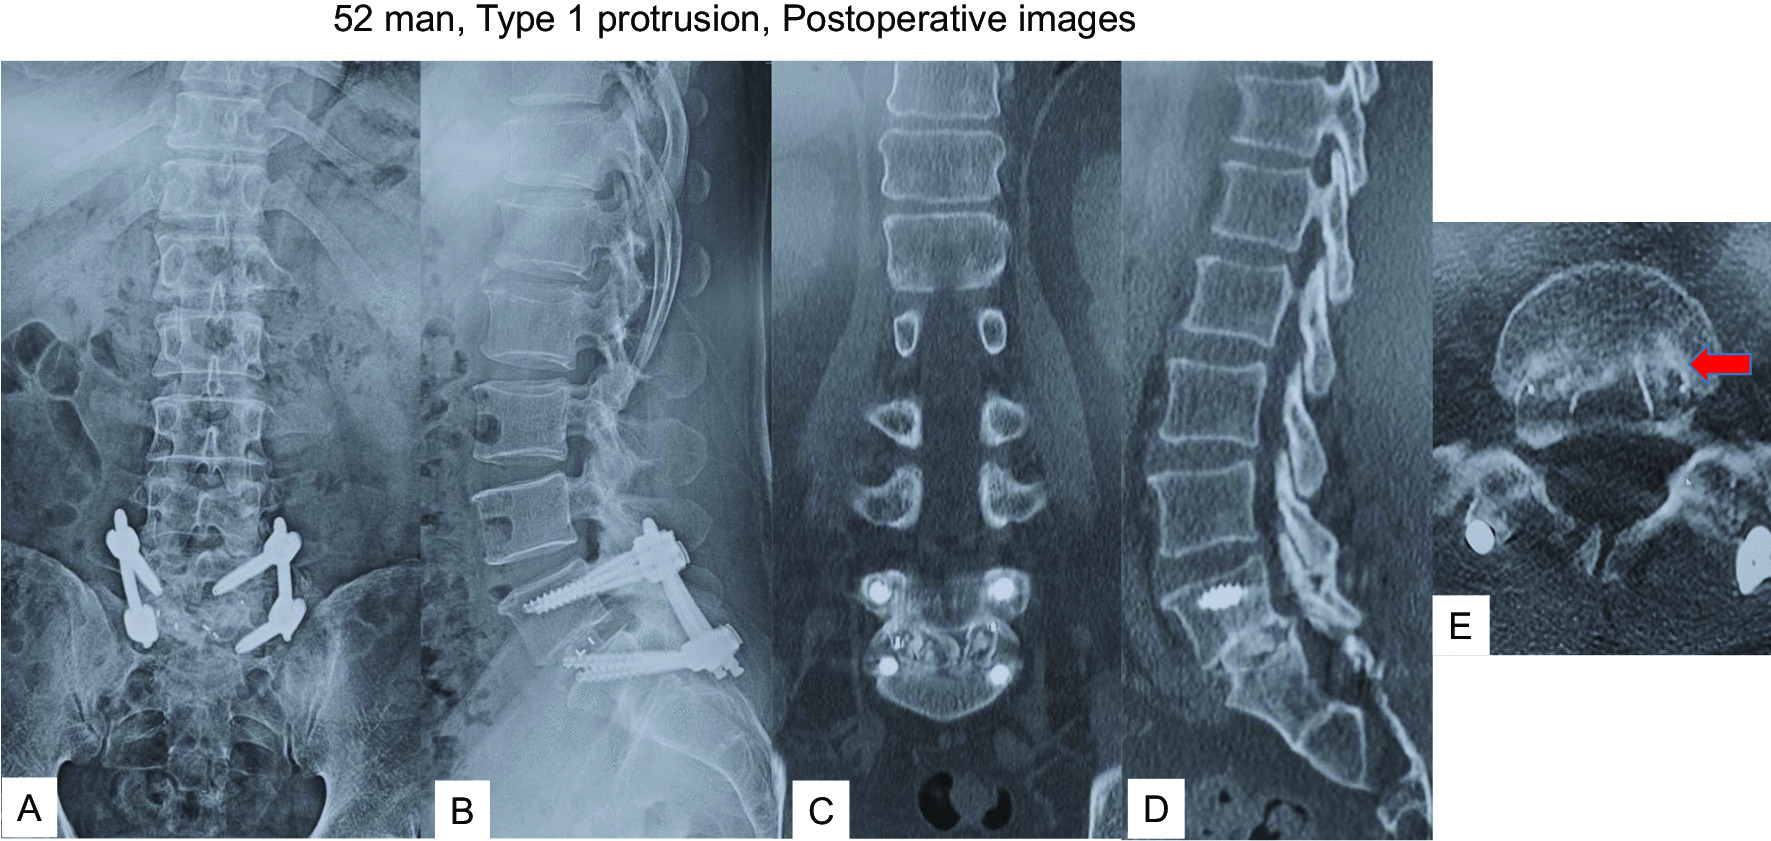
52 man, Type 1 protrusion, postoperative images A Final anteroposterior radiogram, B Final lateral radiogram, C Final coronal reconstruction CT, D Final sagittal reconstruction CT, E Final axial CT at L5/S1 Red arrow shows inserted new cage
